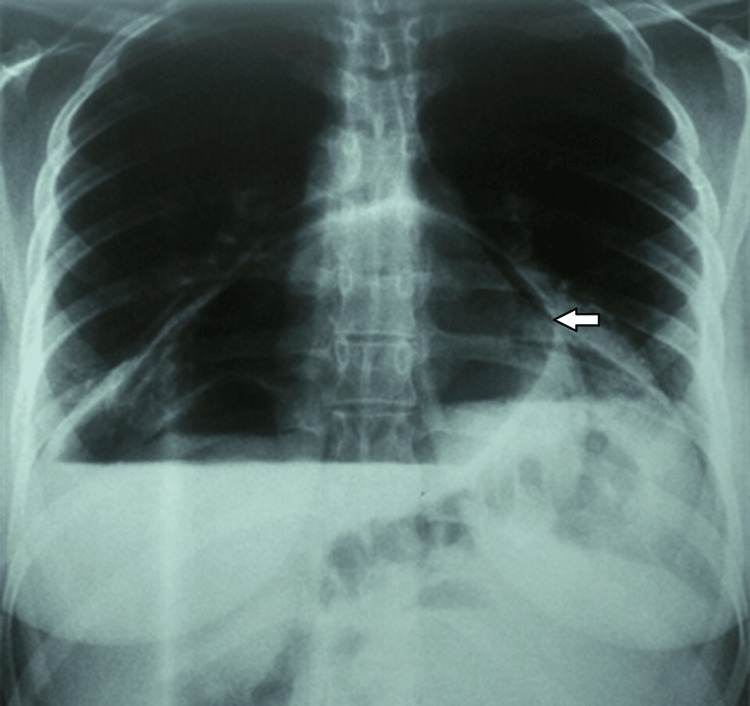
Aeriform image above the diaphragm projecting behind the cardiac opacity on chest X-ray
